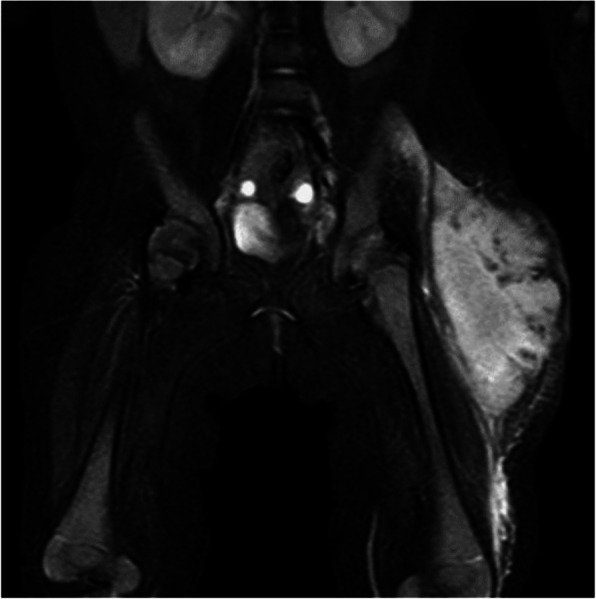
Magnetic resonance imaging: Fat-suppressed T2-weighted image shows a large subcutaneous fluid collection in the left thigh
